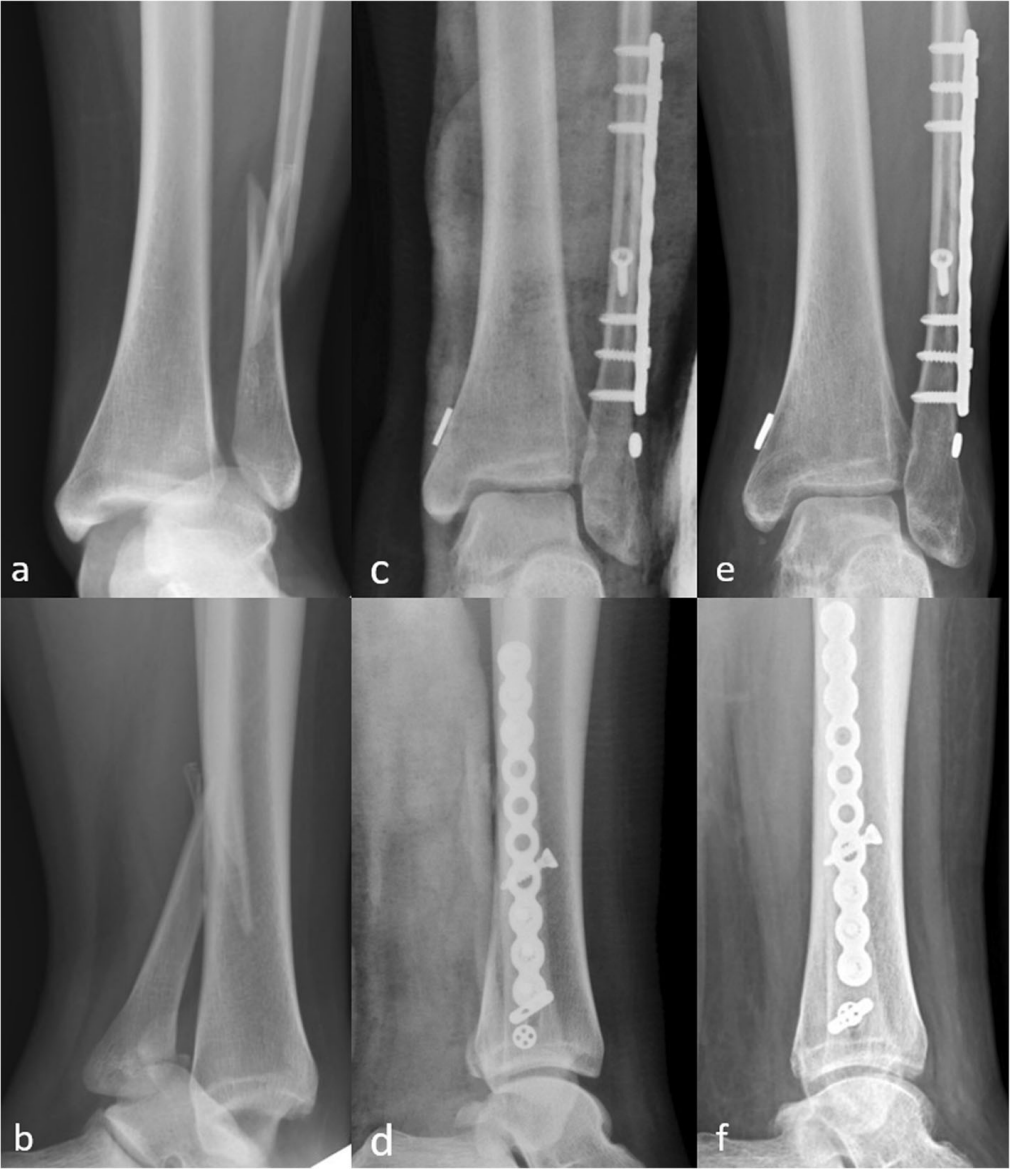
X-ray images of a man experiencing the Weber-C type ankle fracture with syndesmosis injury at 3 timepoints: preoperative (a, b), postoperative (c, d), and final follow-up (e, f)
